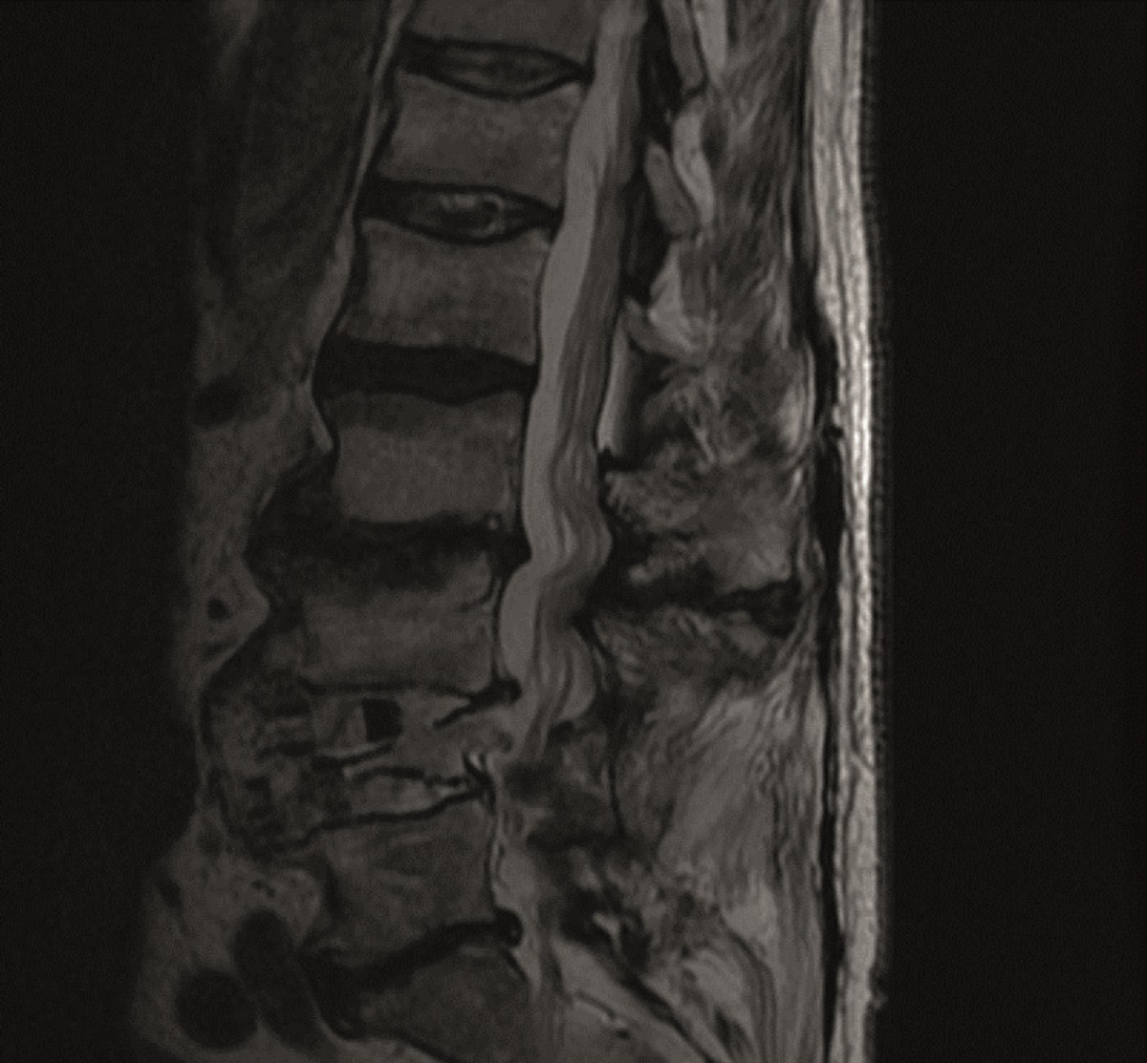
Sagittal T2 Post-operative MRI
